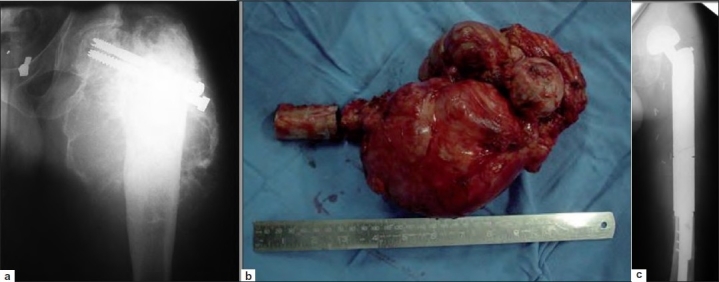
(a) Anteroposterior radiograph of left hip with thigh showing Ewing’s sarcoma with implant *in situ* (b) Photograph showing resected proximal femur with tumor *in situ*. (c) Anteroposterior radiograph of left hip with thigh showing the endoprosthesis *in situ* at 4 years of followup
